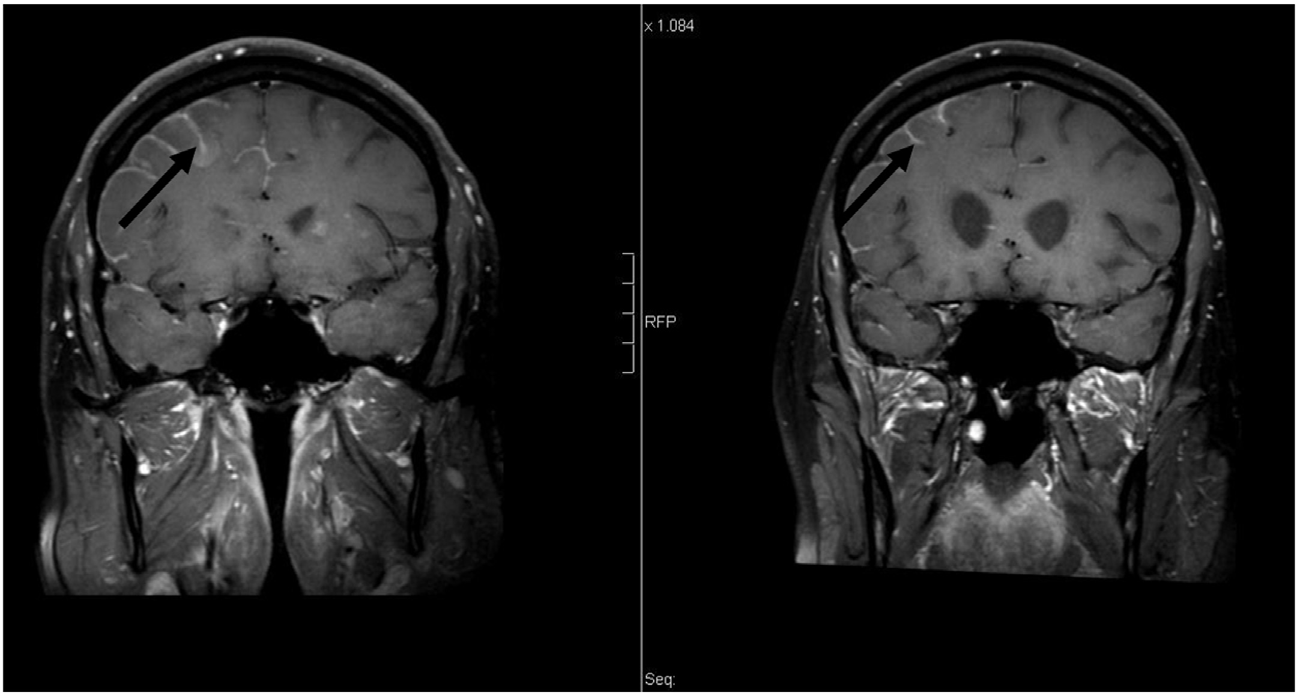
Coronal contrast-enhanced T1-weighted magnetic resonance imaging [left: pre-whole-brain radiotherapy (WBRT); right: 9-month follow-up]: note decrease in leptomeningeal enhancement pre- vs. post-WBRT. In addition, note enlargement of the lateral ventricles on 9-month follow-up scan.
